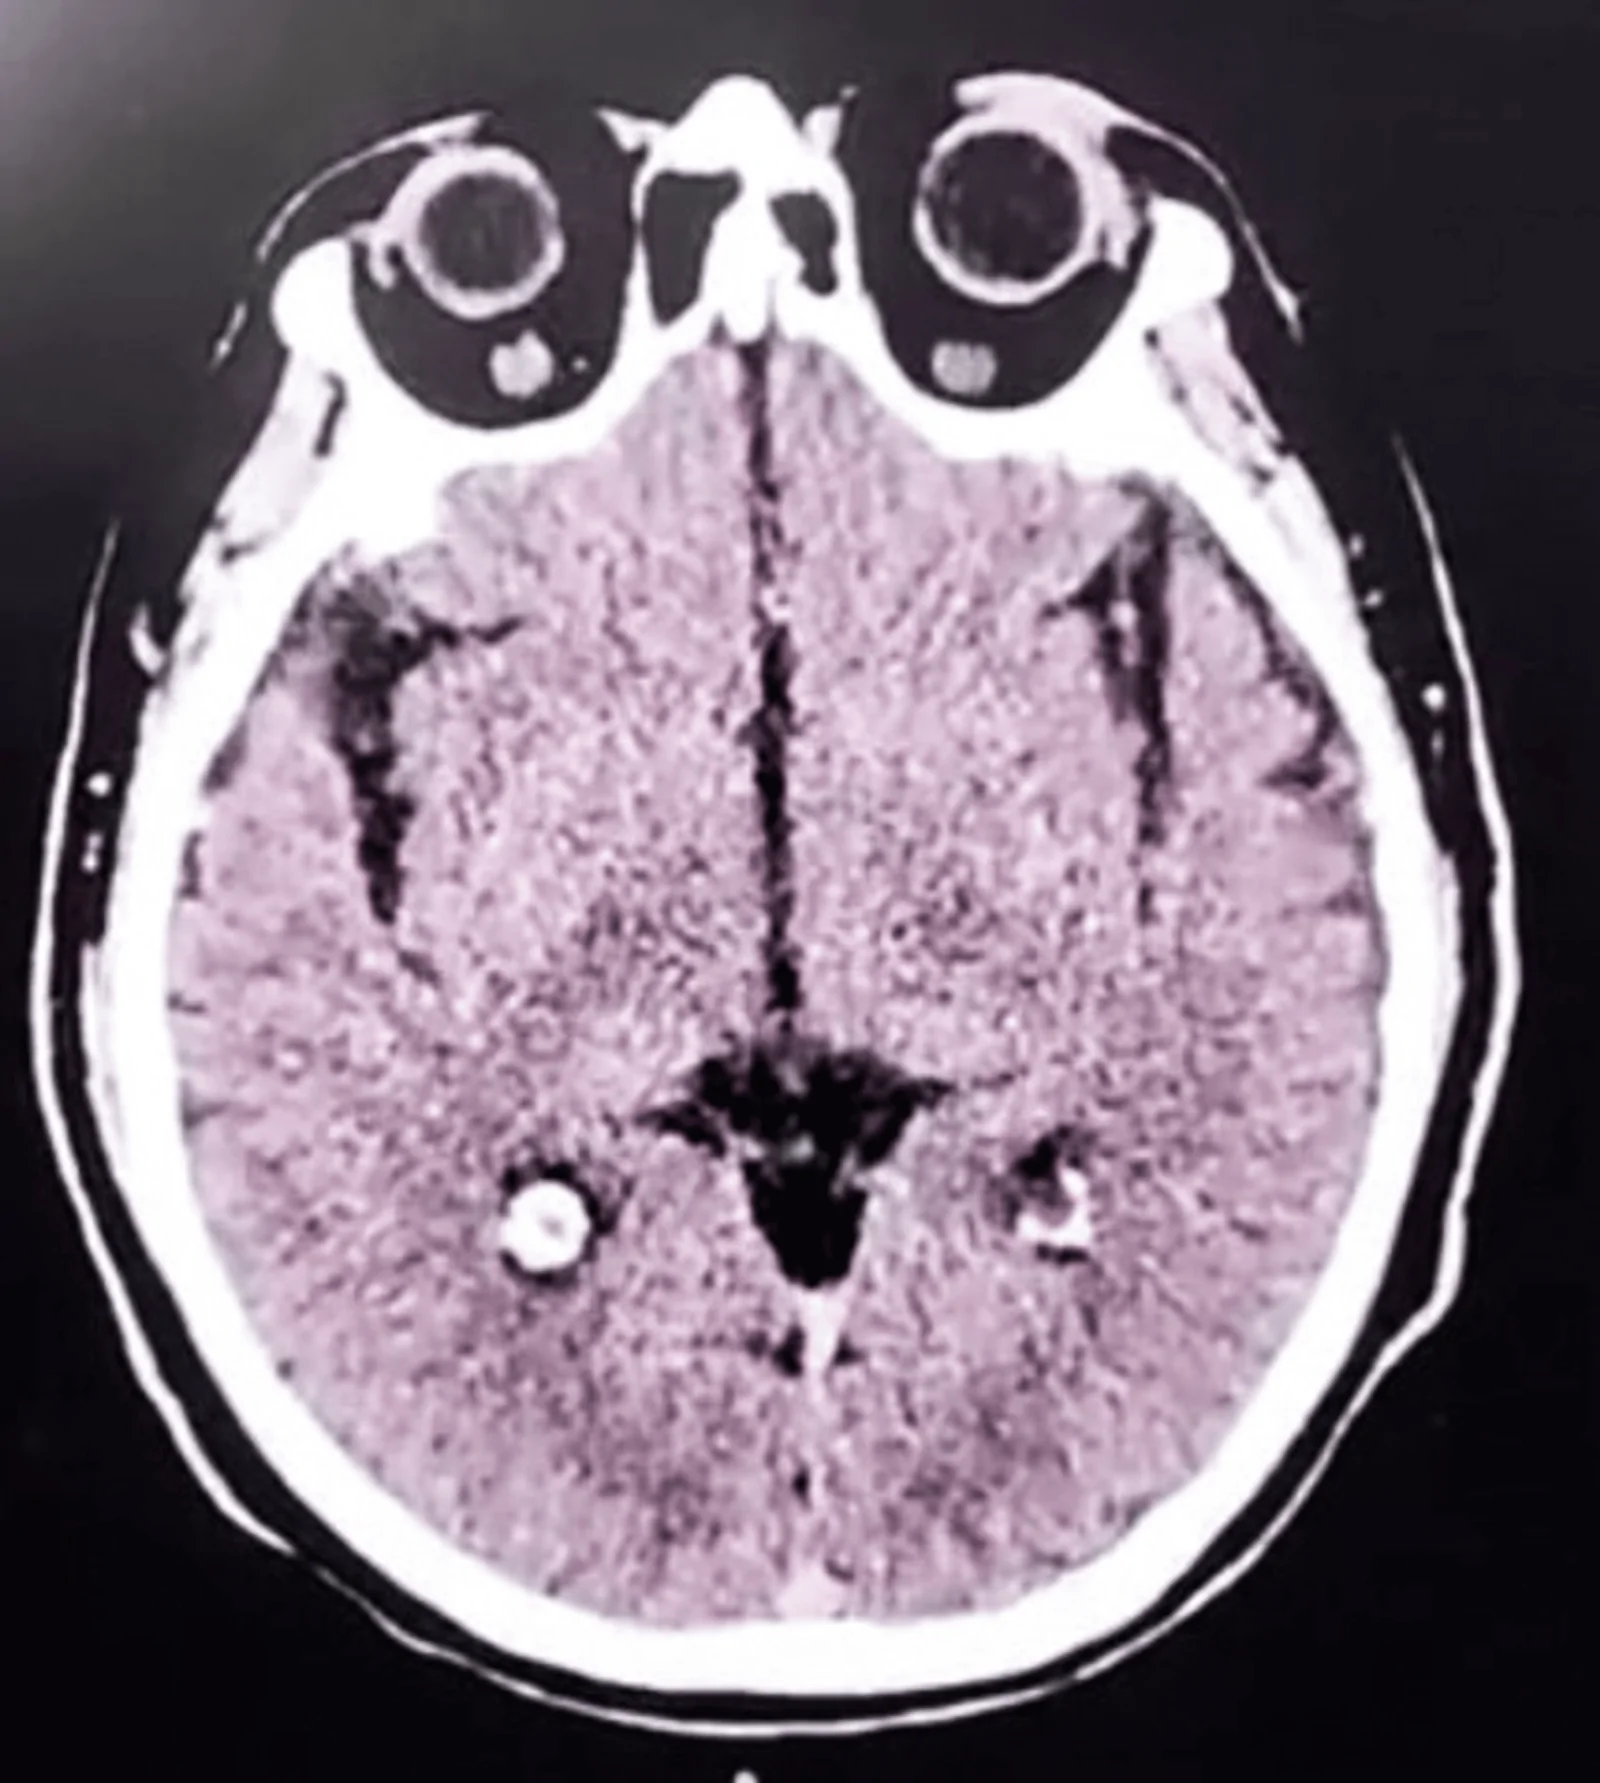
Axial non-contrast CT of the head at the level of the orbits and suprasellar cistern. CT: computed tomography, demonstrating no evidence of acute hemorrhage, mass effect, or other intracranial lesion. Ventricles and sulci are within normal limits for age.
